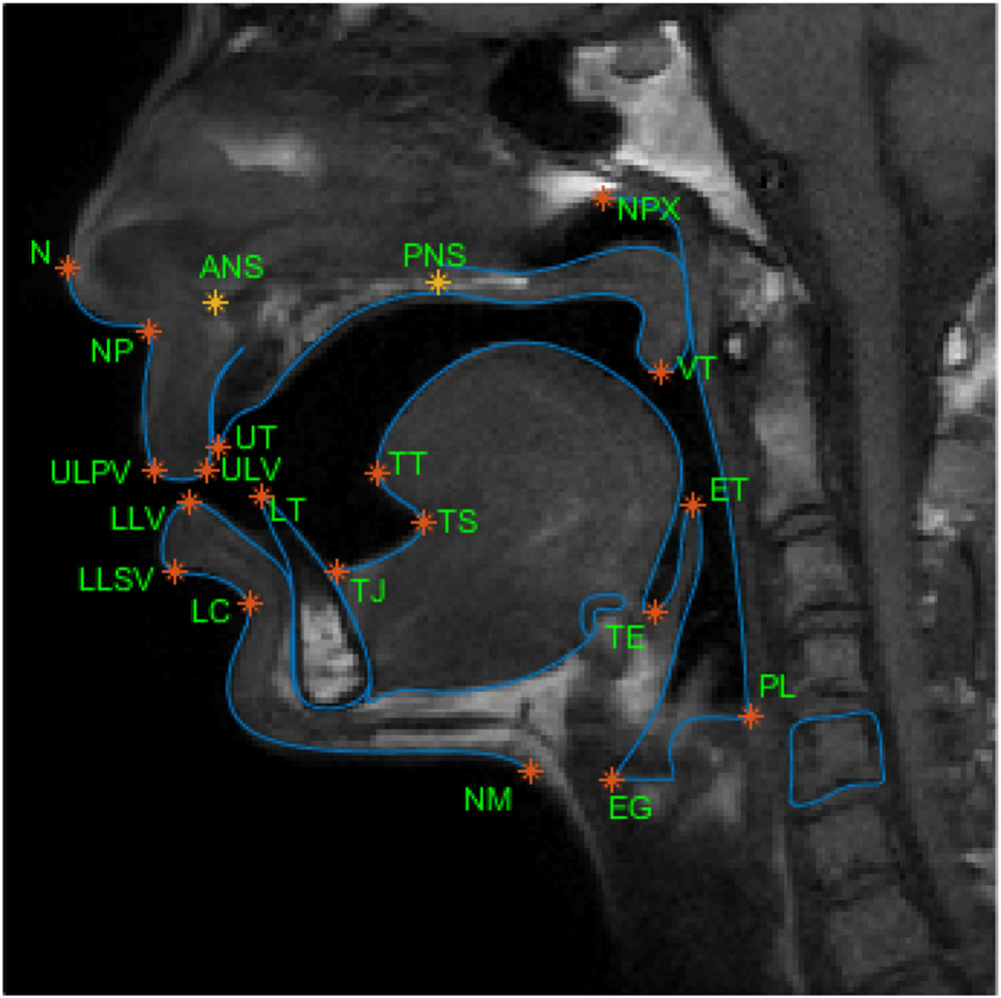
Vocal tract landmarks from MRI image Courtesy of Eslami et al.^82^
